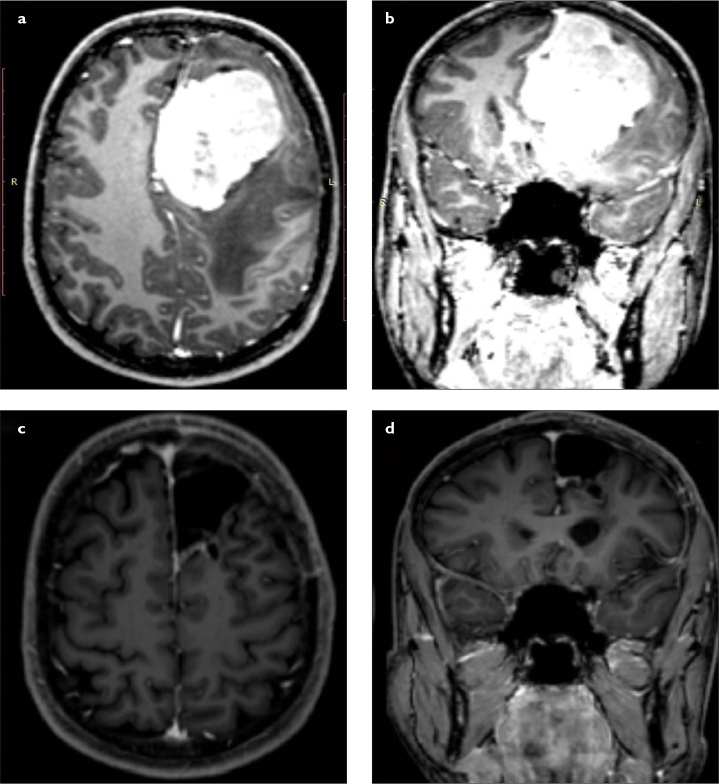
(a) Preoperative axial and (b) coronal MRI scans of a patient with giant left frontal meningioma originating from the falx cerebri. The patient underwent surgical resection using left frontal craniotomy. (c) Postoperative axial and (d) coronal MRI scans confirmed gross total resection. MRI, magnetic resonance imaging.
